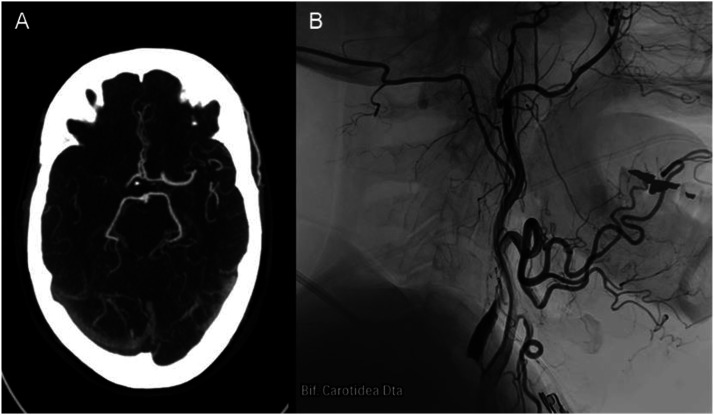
(A) Axial CT angiography depicts M1 occlusion of the right middle cerebral artery and distal segment of right internal carotid artery. (B) Lateral view cervical angiography reveals right internal carotid artery atheroma plaque and post bulbar occlusion.
